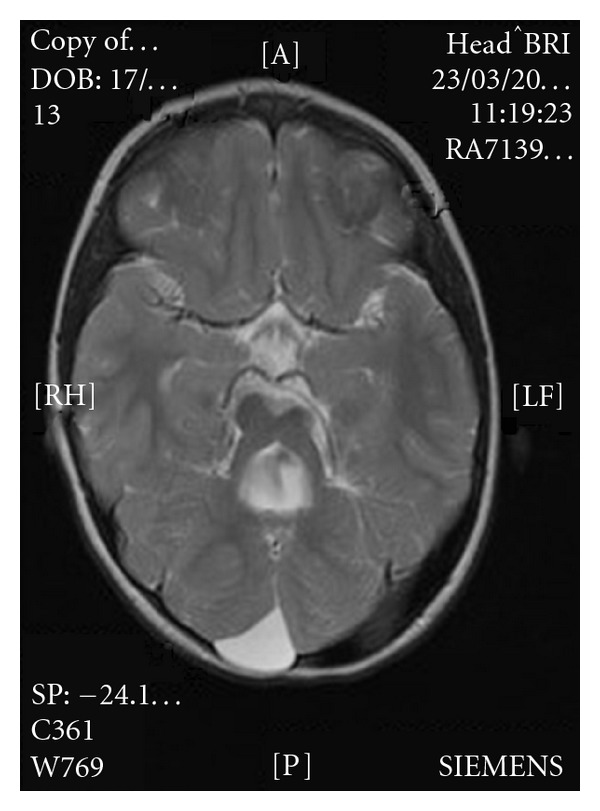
Cranial T2 magnetic resonance image for BZ—this shows an enlarged 4th ventricle with cerebellar vermis hypoplasia and horizontally orientated superior peduncles. There is also a posterior fossa arachnoid cyst.
